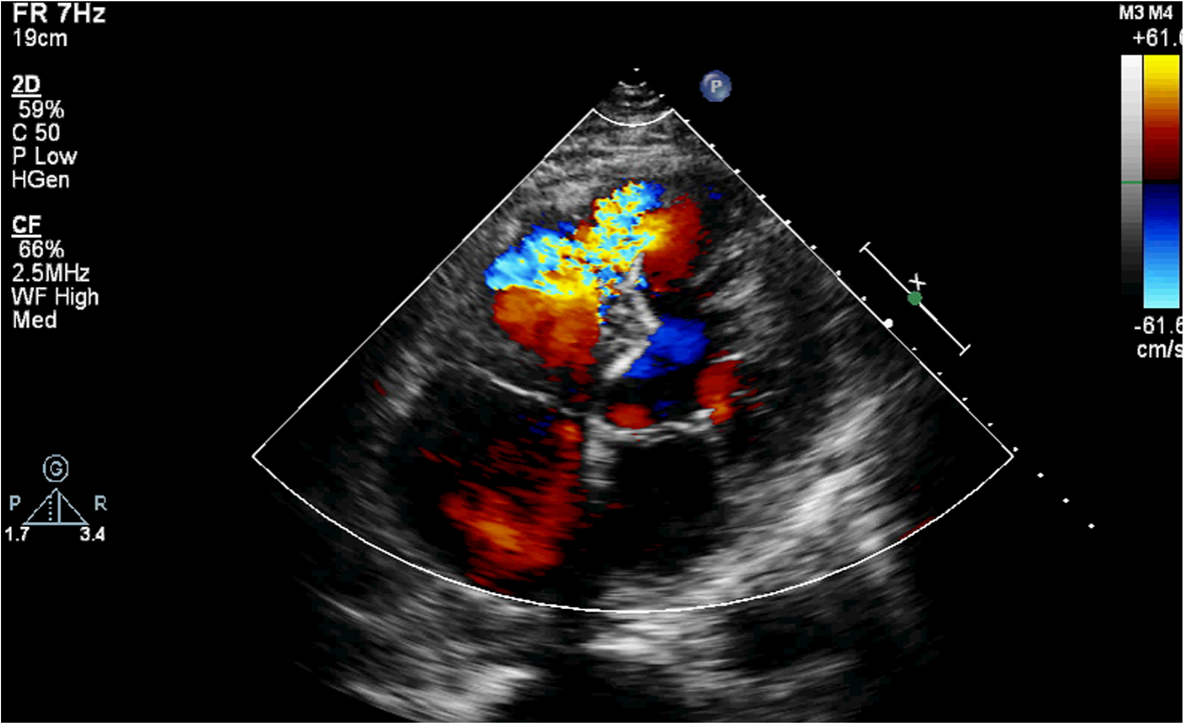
TTE apical 4-chamber view showing bright left-to-right color shunt bundle at the apex of the interventricular septum
